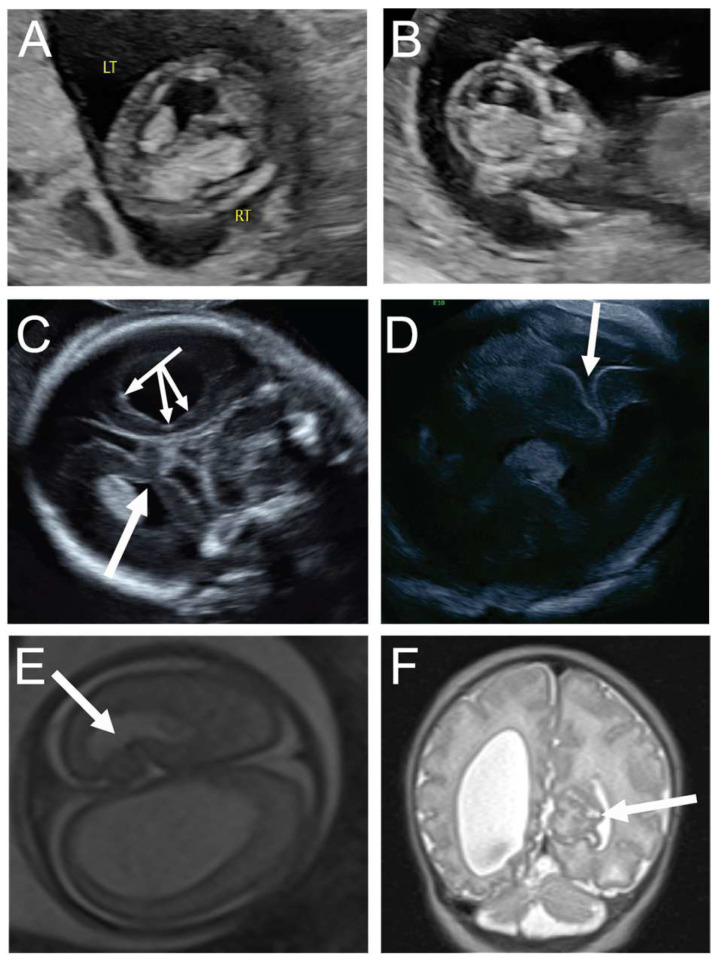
A 31-year-old G3P1 at 13 weeks and 1 day’s gestation was seen for first-trimester screening, and the fetus was noted to have an irregular, asymmetrically enlarged and mottled right choroid plexus, concerning for possible early right intraventricular hemorrhage and ventriculomegaly, note Rt = right side of fetus and Lt = left side of fetus (A,B). Maternal serum infectious and neonatal alloimmune thrombocytopenia workups were negative. Neurosonogram at 20 weeks and 0 days demonstrated the same severe unilateral right ventriculomegaly with stippling along the lining of the right ventricle, consistent with germinal matrix hemorrhage versus heterotopia (triple headed arrow, (C)), with additional findings of right periventricular white matter loss and a contralateral focal area of abnormal folding of the left mesial cortex, concerning for cortical dysplasia or polymicrogyria (single arrow, (C)). Fetal MRI confirmed right unilateral ventriculomegaly with evidence of right germinal matrix and intraventricular hemorrhage, as well as cortical abnormality in the left mesial occipital lobe (arrow, (E)). The mother opted to continue the pregnancy and declined amniocentesis. Ultrasound at 26 weeks and 5 days’ gestation showed progressive right ventriculomegaly with midline shift and continued prominence of contralateral cortical dysplasia, with possible progression to schizencephaly (arrow, (D)). She was delivered via cesarean at 36 weeks in the setting of preterm labor. Postnatal MRI demonstrated worsening of the cortical maldevelopment in the left mesial occipital lobe (arrow, (F)), a few scattered foci of periventricular nodular heterotropia, and abnormal vertical and shallow appearance of the right sylvian fissure. At two years of age, the child was meeting developmental milestones.
